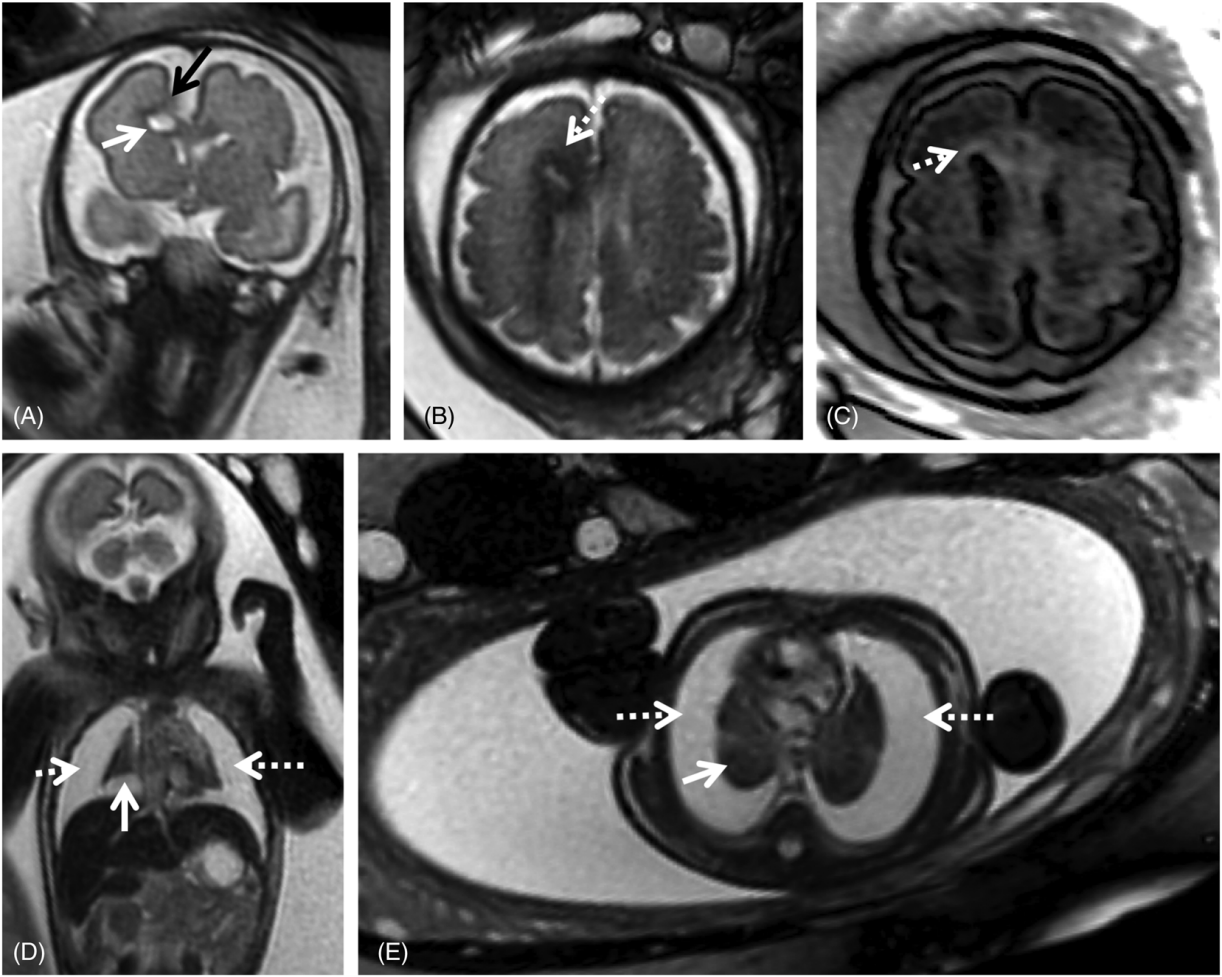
Magnetic resonance imaging of fetus referred at 31 gestational weeks for hydrothorax and suspected frontal brain lesion. On T2WI over the fetal brain (A,B) it is possible to identify a destructive lesion of the right frontal lobe, with focal parenchymal loss (A, black arrow), with associated intraparenchymal white matter cystic lesion (A, white arrow) and altered signal of the surrounding parenchyma, with T2 hypointensity (B, white dashed arrow) and high signal on T1‐weighted FLAIR imaging (C, white arrow), suggesting gliotic changes. There are extensive bilateral pleural effusions (D, E, dashed white arrows), with severe reduction of the lung volume (D, E, white arrows)
